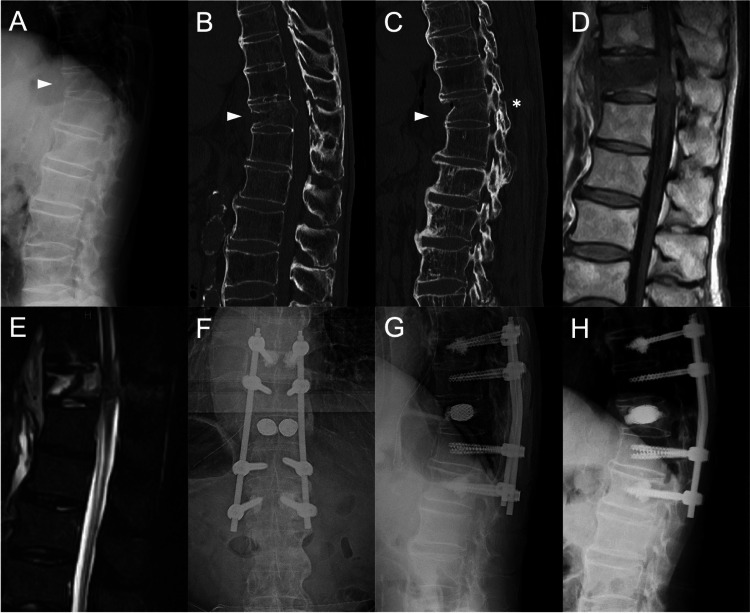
Case A. Preoperative radiographs; B. Median sagittal CT image; C. Paramedian sagittal CT image; D. T1-weighted MRI image; E. STIR MRI image; F. Anterior-posterior radiographs after one week of surgery; G. Lateral neutral radiographs after one week of surgery; H. Lateral neutral radiographs after six-month surgery. CT: computed tomography; STIR: short inversion-time inversion recovery; MRI: magnetic resonance imaging
